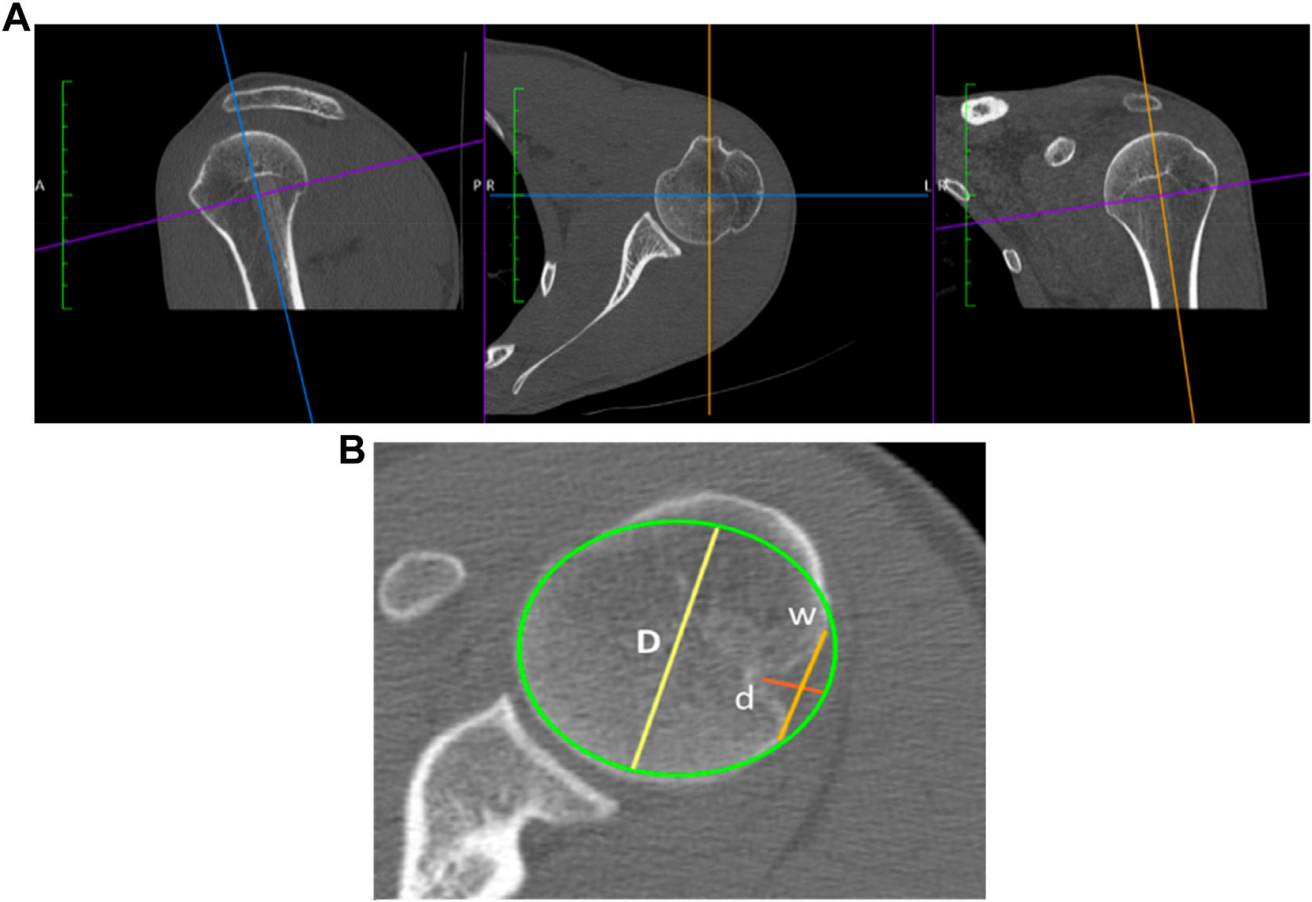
Hill-Sachs lesion depth and width measurement. (A) The CT-scan was reformatted on the axial plan of the humerus (perpendicular to the axis of the diaphysis). (B) Measurement of the Hill-Sachs lesion (*D*, diameter of the circle; *d*, depth; *w*, width). *CT*, computed tomography.
